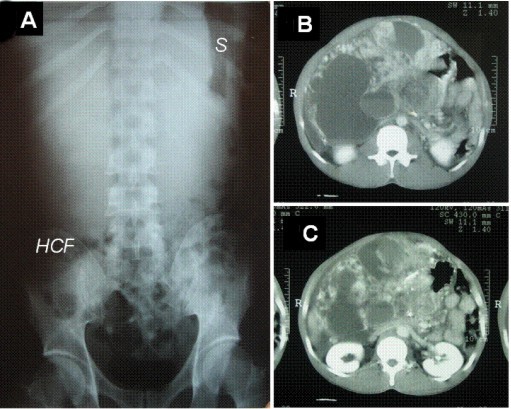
Radiology: A) Abdominal X-ray showing a diffuse opaque area in upper abdomen without a gastrointestinal gas shadow, hepatic colon flexure was dislocated downward (HCF), and the stomach (S) to the left side.B and C). Abdominal computed tomography scan showing heterogenous mass occupying most part of the abdominal cavity.
